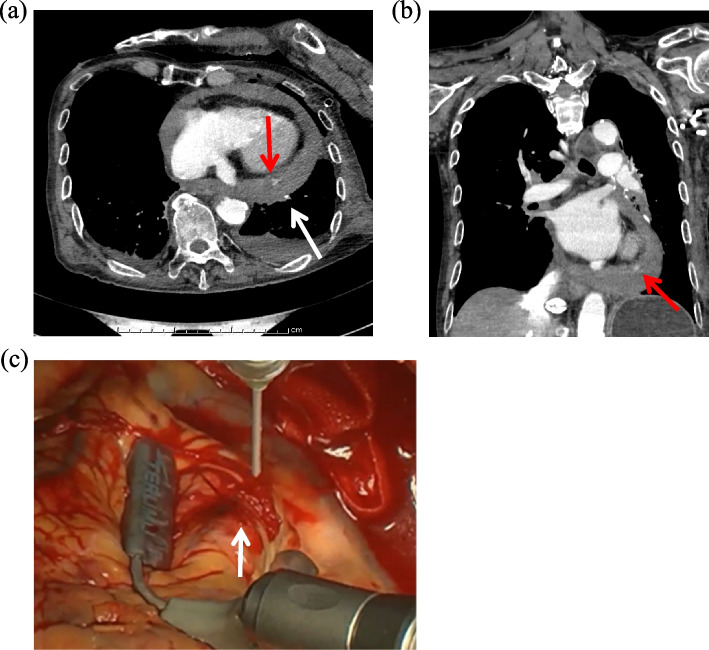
a Postoperative chest computed tomography in axial section. A postoperative enhanced chest computed tomography reveals hemorrhagic cardiac tamponade and extravascular leakage of contrast agent (red arrow) is shown in the pericardium. The staple line dividing the interlobar fissure is shown by the white arrow. b Postoperative chest computed tomography in coronal section. A postoperative enhanced chest computed tomography in coronal section reveals that the hemorrhagic tamponade and extravascular leakage of contrast agent (red arrow) is located at the apex of the heart. c Intraoperative image of coronary artery repair. A laceration is noted on the posterolateral branch of the left circumflex artery and blood was spurting out (white arrow)
